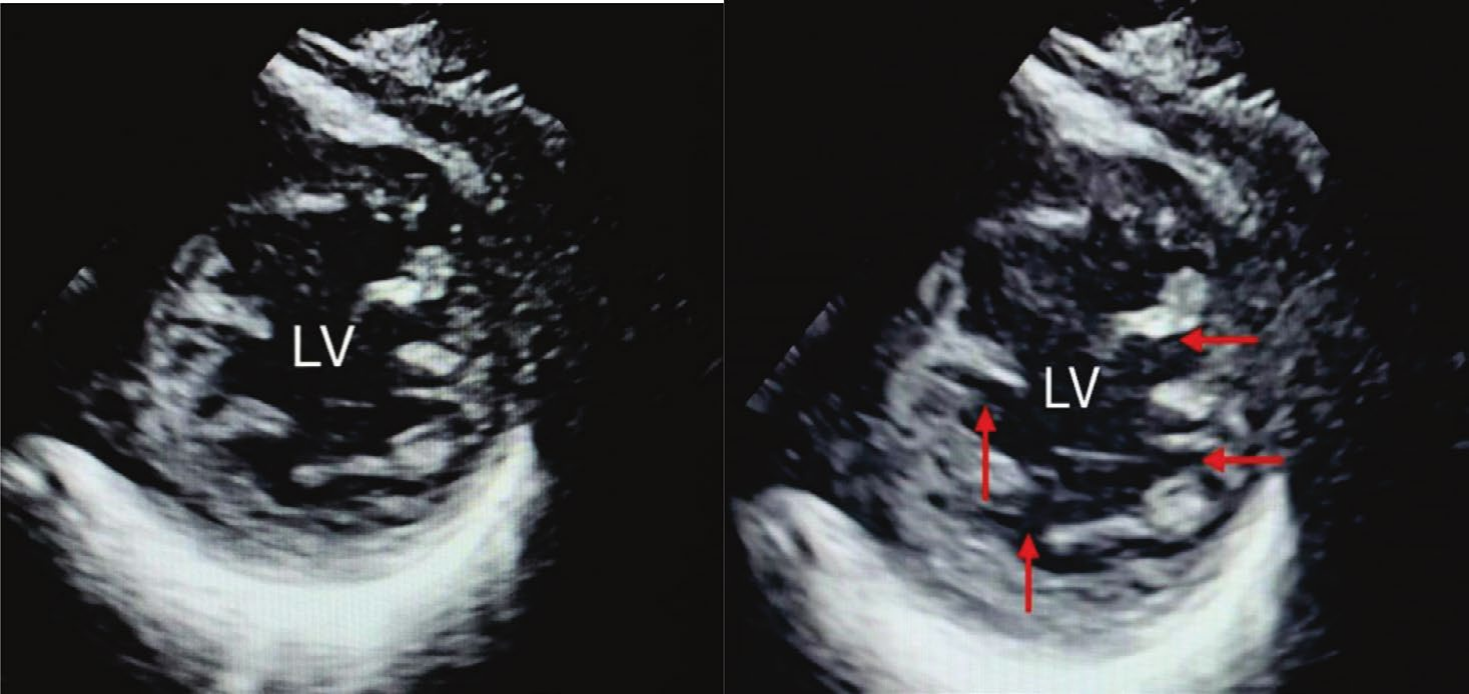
Transthoracic echocardiography: Parasternal short axis view: Prominent left ventricle (LV) trabeculations with deep recesses. The ratio of thickness of non‐compacted:compacted layer > 2:1.
